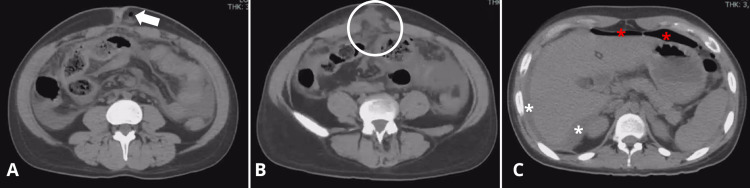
Non-contrast axial computed tomography of the abdomen showing an abdominal wall defect A: Axial CT image demonstrates a fluid collection and the presence of gas within the subcutaneous tissue at the level of the umbilical scar (white arrow). Additionally, free fluid is observed in the interloop spaces and along the left paracolic gutter. B: The image reveals a tract establishing communication between the intra-abdominal free fluid and the subcutaneous tissue of the abdominal wall (white circle), suggestive of a fistulous connection or abscess rupture. C: A water-density image shows perihepatic free fluid (white asterisks), and a hypodense area in the prehepatic region is suggestive of free intraperitoneal air (red asterisks), indicating possible bowel perforation.
